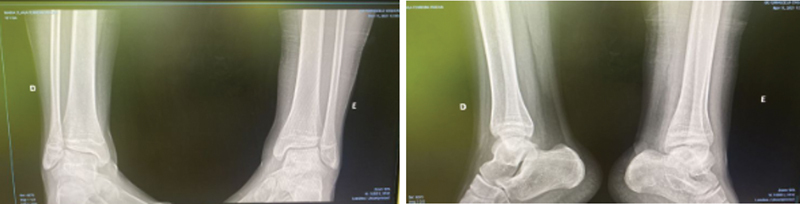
Ankle X-ray. Source: Service collection.
